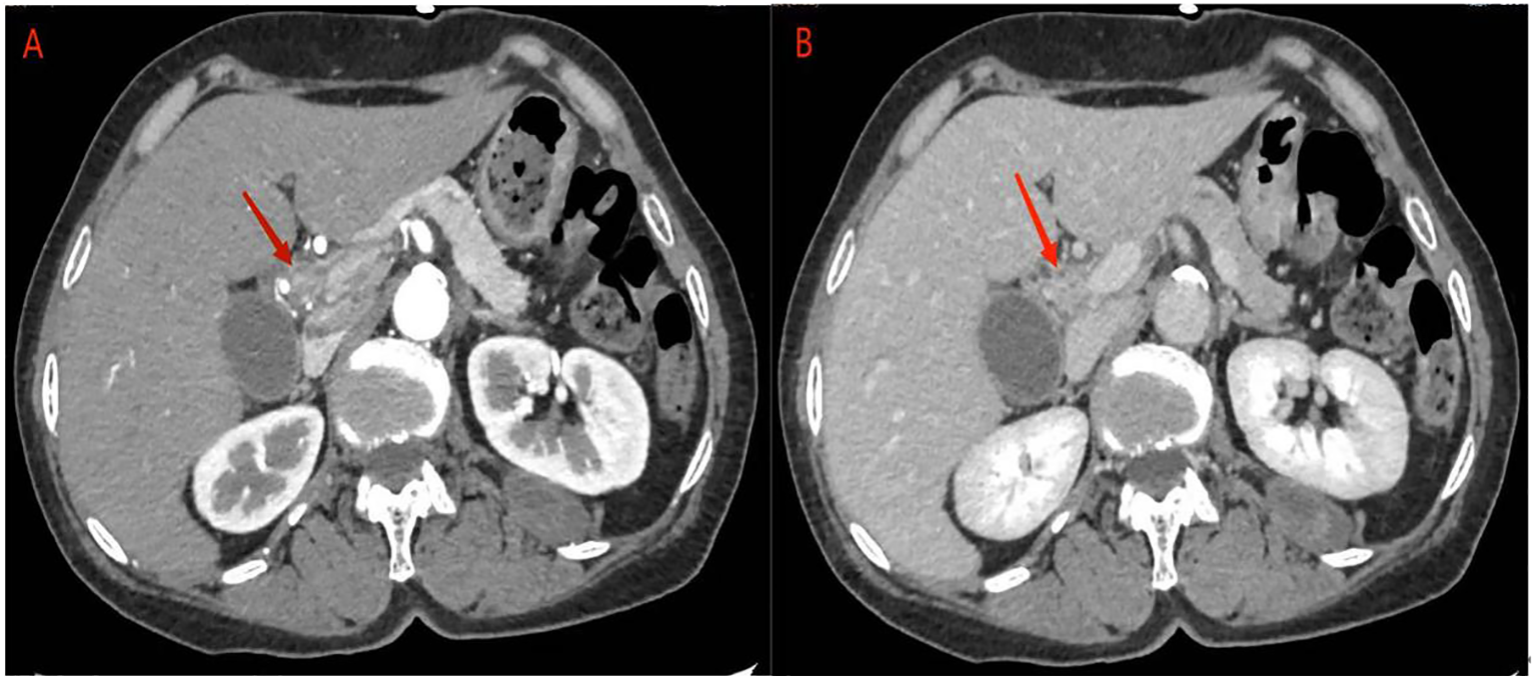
abdominal contrast-enhanced CT: (A) the contrast-enhanced scan of the tumor showed significant enhancement, corresponding to narrowing of the bile duct lumen. (B) In the delayed phase, tumor enhancement may be reduced.
